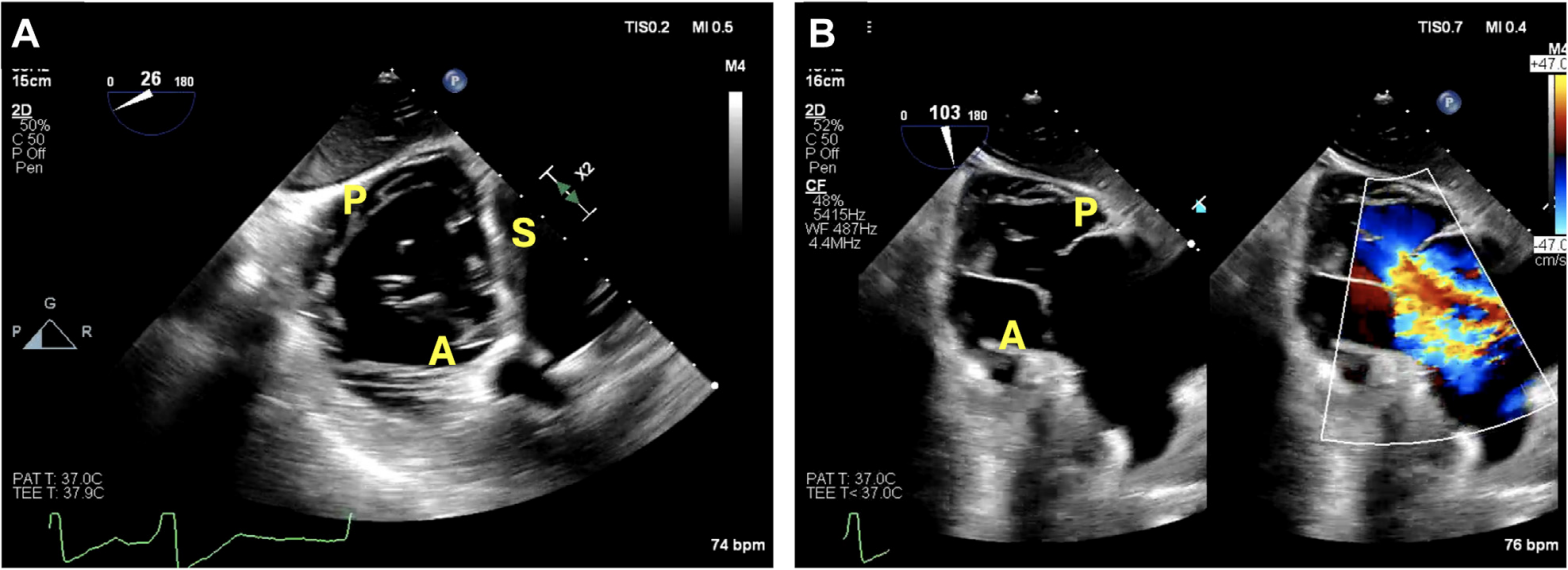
Baseline TEE Imaging (A) Demonstrates short axis view of the tricuspid valve via a gastric image on transesophageal echocardiography. The 3 leaflets, septal (S), anterior (A), and posterior (P), are visualized along with the coaptation gap. (B) The long-axis view of the right ventricle on gastric images shows the posterior and anterior leaflet again with the large coaptation gap along with torrential tricuspid regurgitation.
